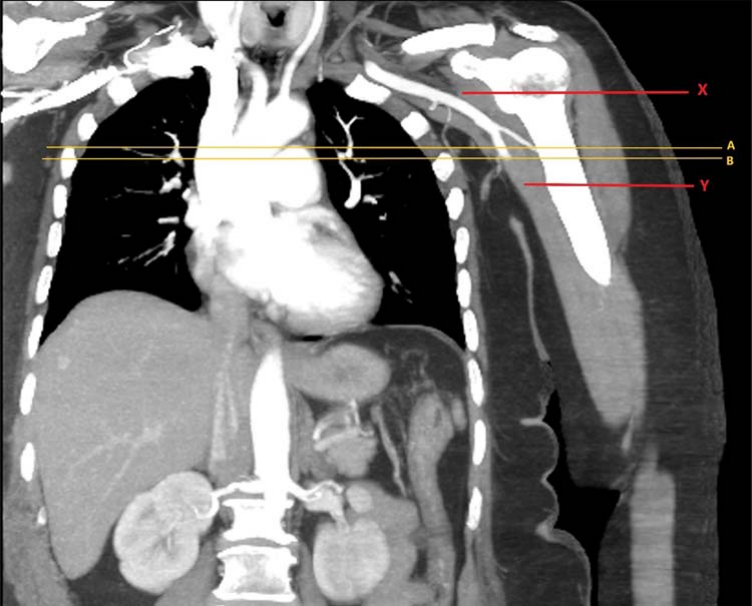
CTA of left upper limb in the coronal plane. X—axillary artery with contrast showing flow as evident in transverse imaging (see Fig. 3), patent in the transverse plane marked A. Flow ceases approximately at the level of the plane marked B. Y—axillary artery occluded as shown with contrast absent.
